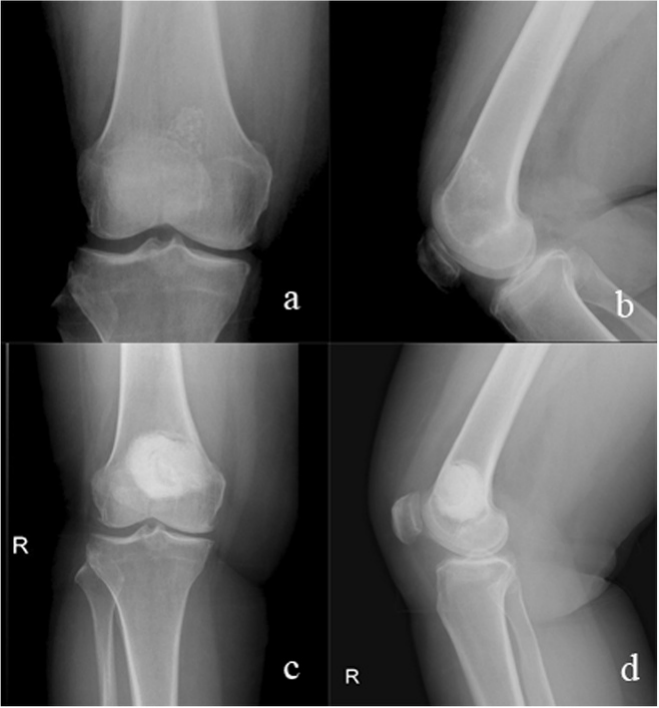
Radiographic findings in low grade chondrosarcoma of distal femur. (a, b) A 52-year-old female patient with a low-grade chondrosarcoma in the right distal femur. (c, d) Radiographs after 48 months from the surgery.
